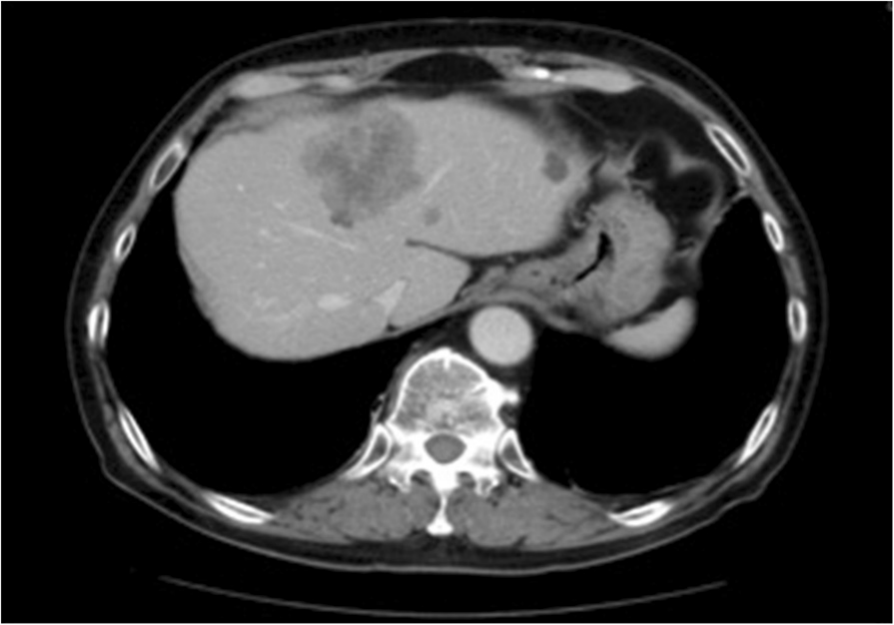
Computed tomography of liver metastasis. Abdominal CT showing shadows on liver at S4 and S8.
